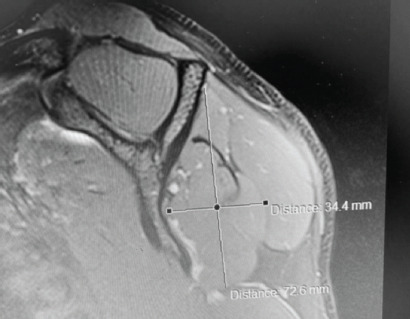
MRI showing complete resolution of the cyst and recovery of infraspinatus muscle bulk.
